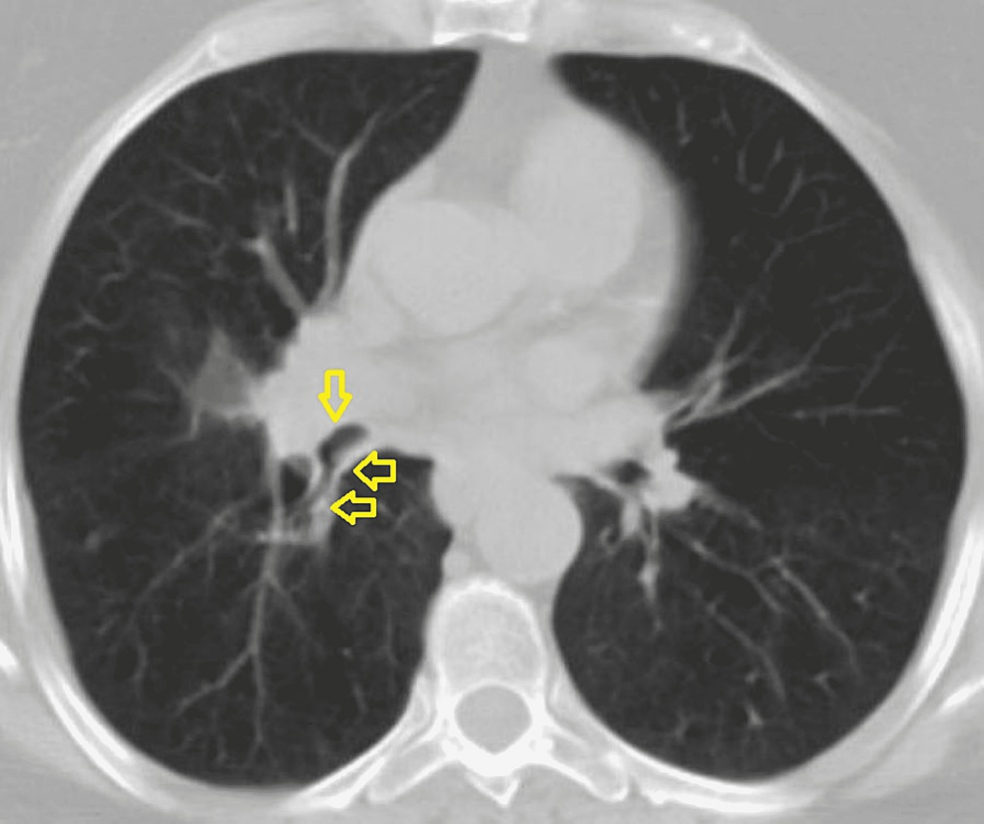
CT scan of the chest showing right middle bronchus obstruction
